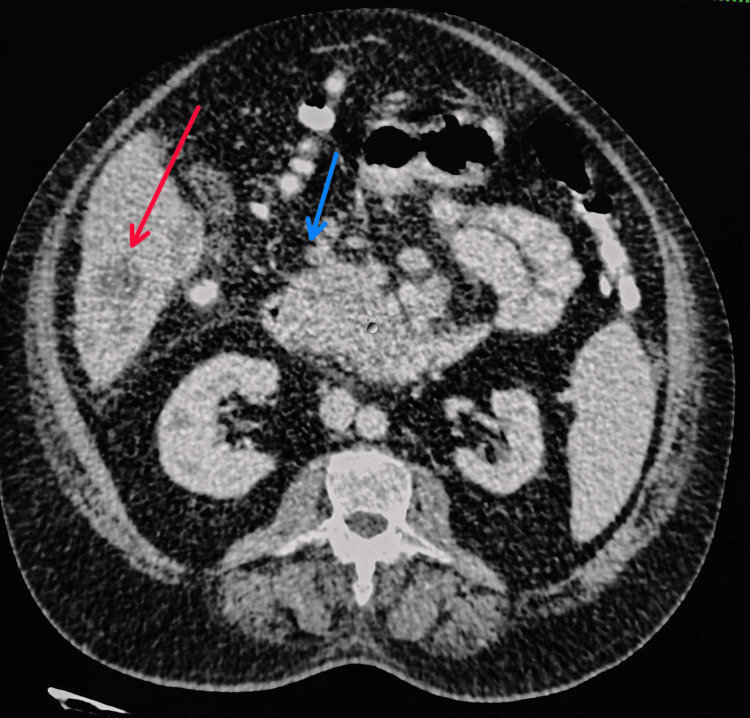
CECT abdomen showing multiple peripherally enhancing hepatic lesions (red arrow) and a hypodense pancreatic uncinate lesion (blue arrow) CECT: contrast-enhanced computed tomography
